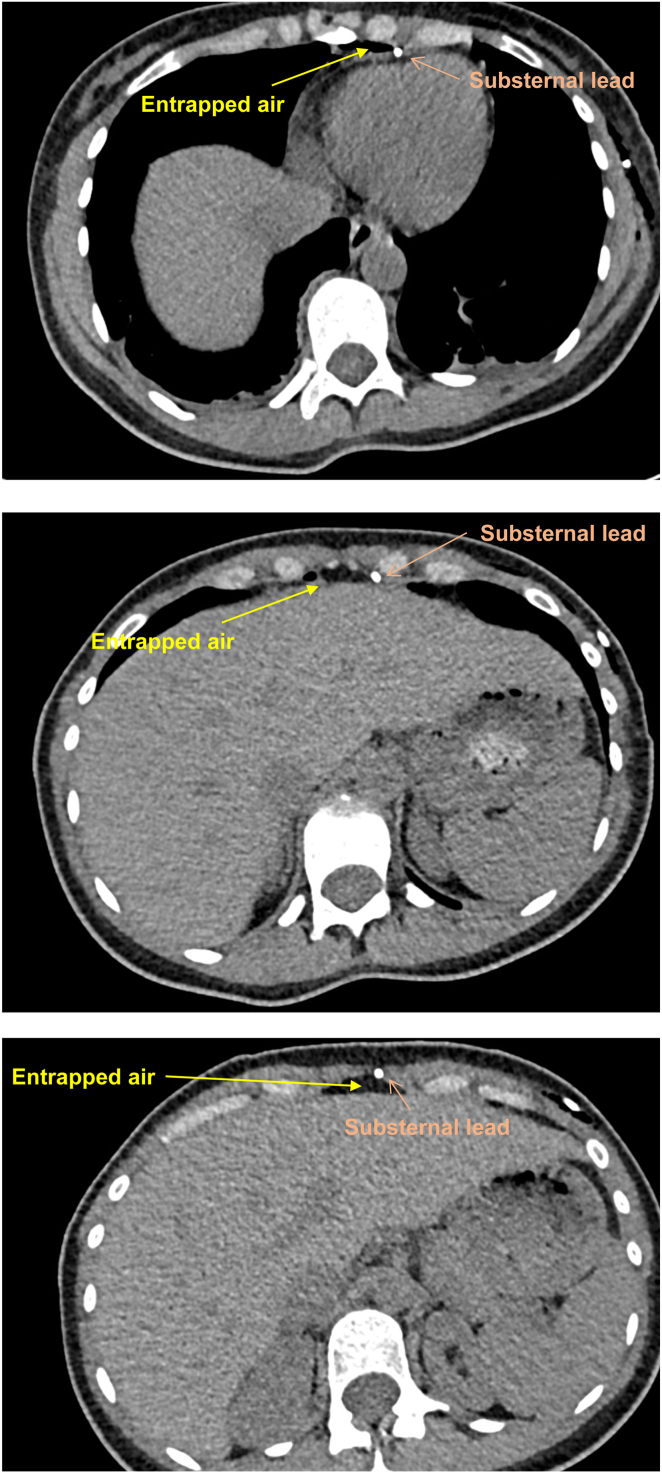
Chest computed tomography scan confirmed the presence of entrapped air surrounding the substernal lead as well as in the tunnel from the subxiphoid pocket.
